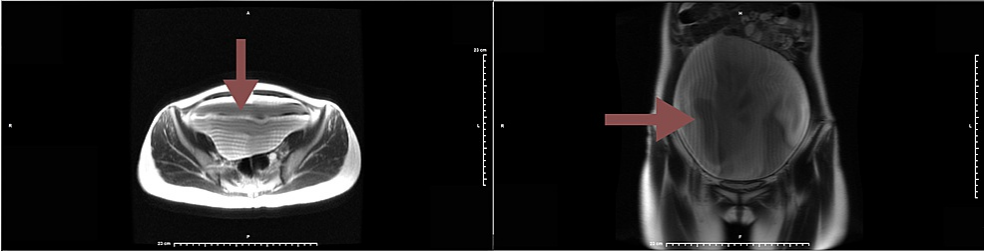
MRI transverse (left) and MRI coronal (right) – midline cystic lesion (red arrow)
